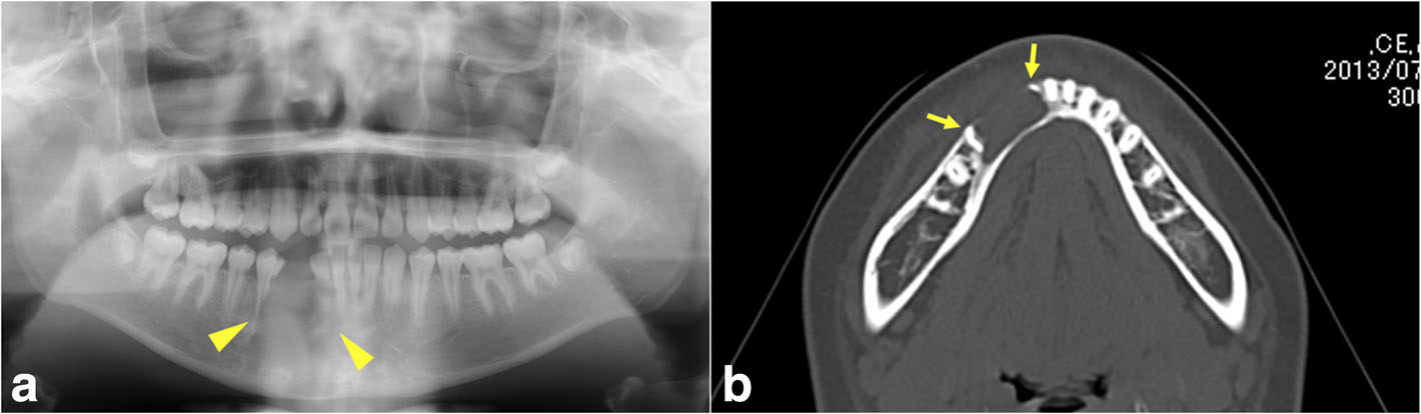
a Panoramic tomography showed the resorption of the root of tooth 42 and medial side of the root of tooth 44 (*arrow heads*). b Computed tomography revealed a well- circumscribed 3-cm osteolytic lesion in the right mandible that eroded the buccal cortical plate and resorbed the roots of tooth 42 and tooth 44 (*arrows*)
